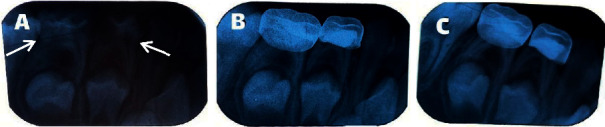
(a) Pretreatment radiography, (b) radiographic success in 6-months follow-up, (c) radiographic success in 12-months follow-up (treated teeth are marked with arrows; the second molar is intended for evaluation).
